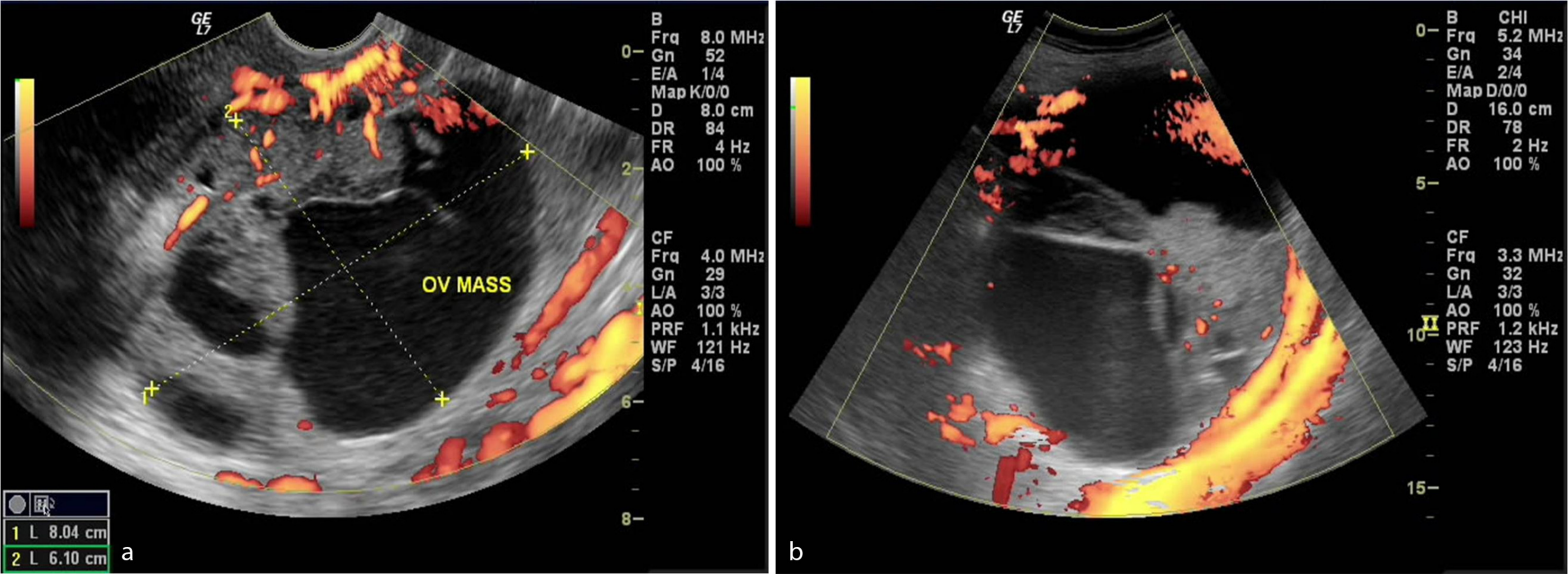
(a and b) Ultrasound with power Doppler imaging shows bilateral complex ovarian masses. OV, ovarian.
